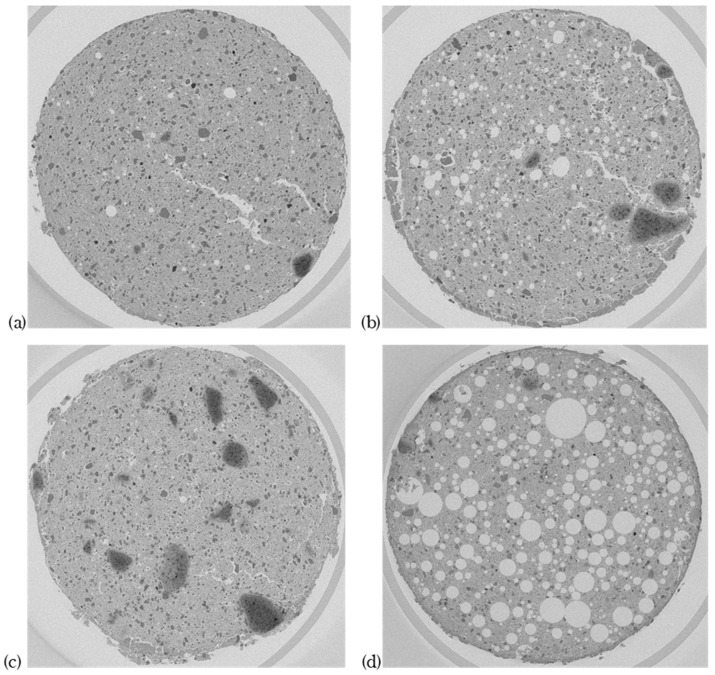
Raw images from X-ray tomography for the mix designs C = 12% w/w_L_ = 1.6 M = 0% (a), C = 12% w/w_L_ = 1.6 M = 50% (b), C = 18% w/w_L_ = 2 M = 0% (c), and C = 18% w/w_L_ = 2 M = 50% (d).
